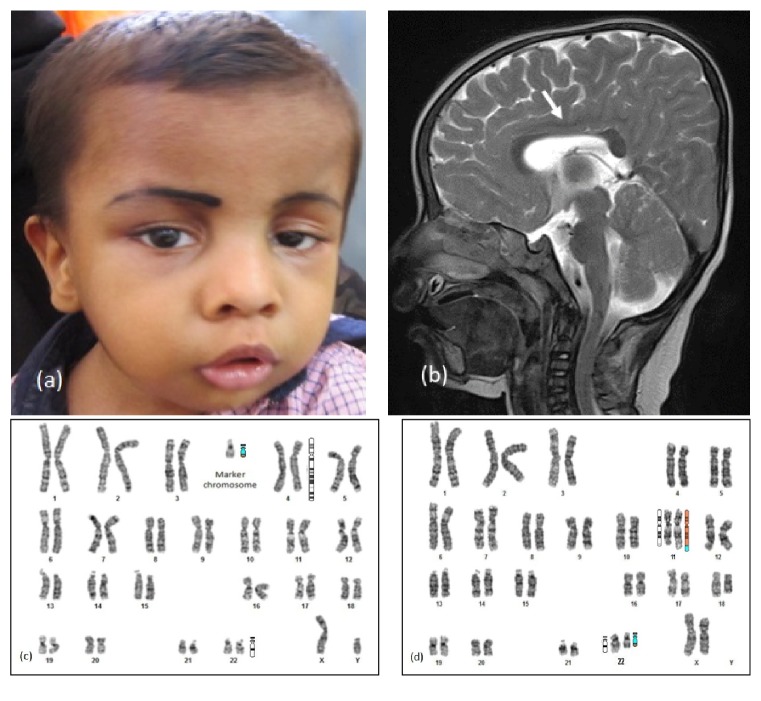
Proband 1 with Emanuel syndrome: (a) phenotype with unilateral left ptosis, microretrognathia, (b) T2 weighted magnetic resonance imaging of brain (sagittal) showing hypoplastic body of corpus callosum (arrow), (c) karyotype and ideogram showing marker chromosome, and (d) karyotype and ideogram of mother showing t(11; 22)(q24; q12).
